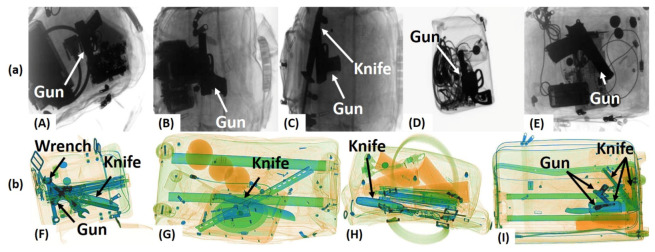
((A)–(I)) Baggage X-ray scans containing extremely cluttered and overlapping contraband items such as *guns*, *knives* and *wrenches*. Row (a) shows the scans from the GDXray [15] dataset and row (b) shows the scans from the SIXray [14] dataset.
